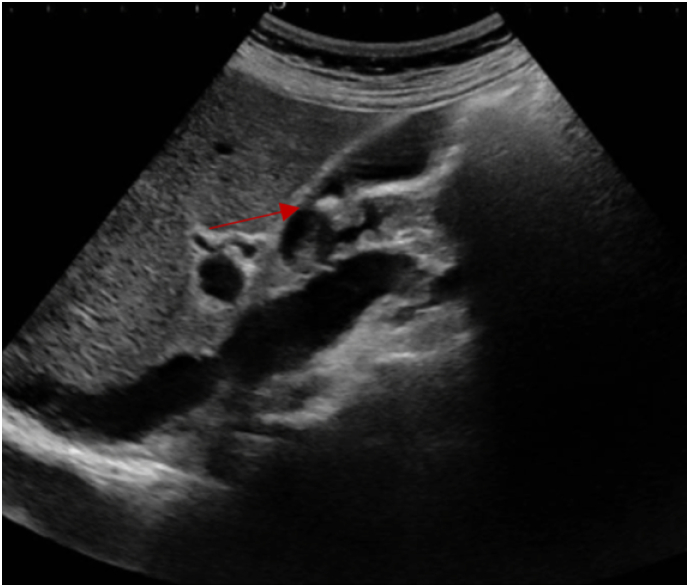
Gallbladder ultrasound done at The Peripheral Hospital. Red arrow pointing to the fold that was initially considered a stone at the peripheral hospital. (For interpretation of the references to colour in this figure legend, the reader is referred to the web version of this article.)
